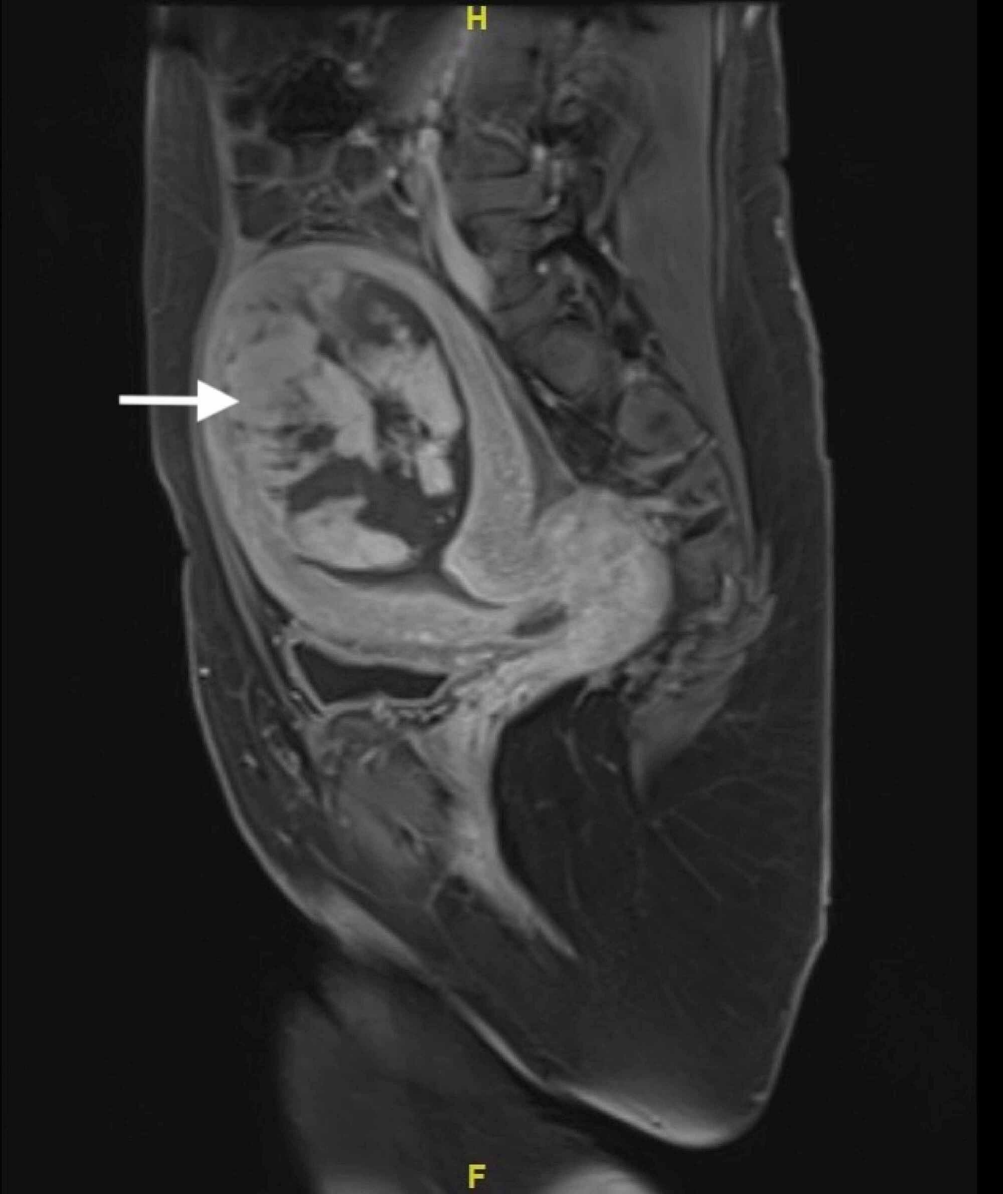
MRI showing an intra-cavity soft tissue mass measuring 6.5 cm.
